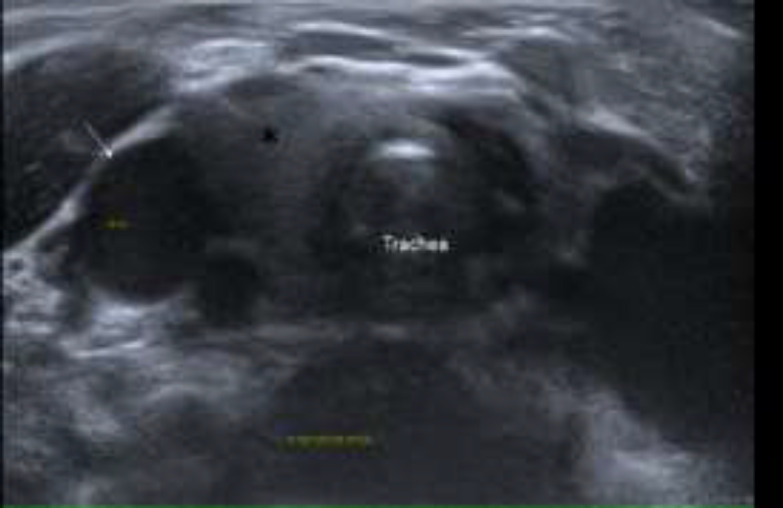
Ultrasound scan of the neck in axial view taken at suprasternal level shows sill-defined soft tissue in between right internal jugular vein (white arrow) and trachea (*)at rest
